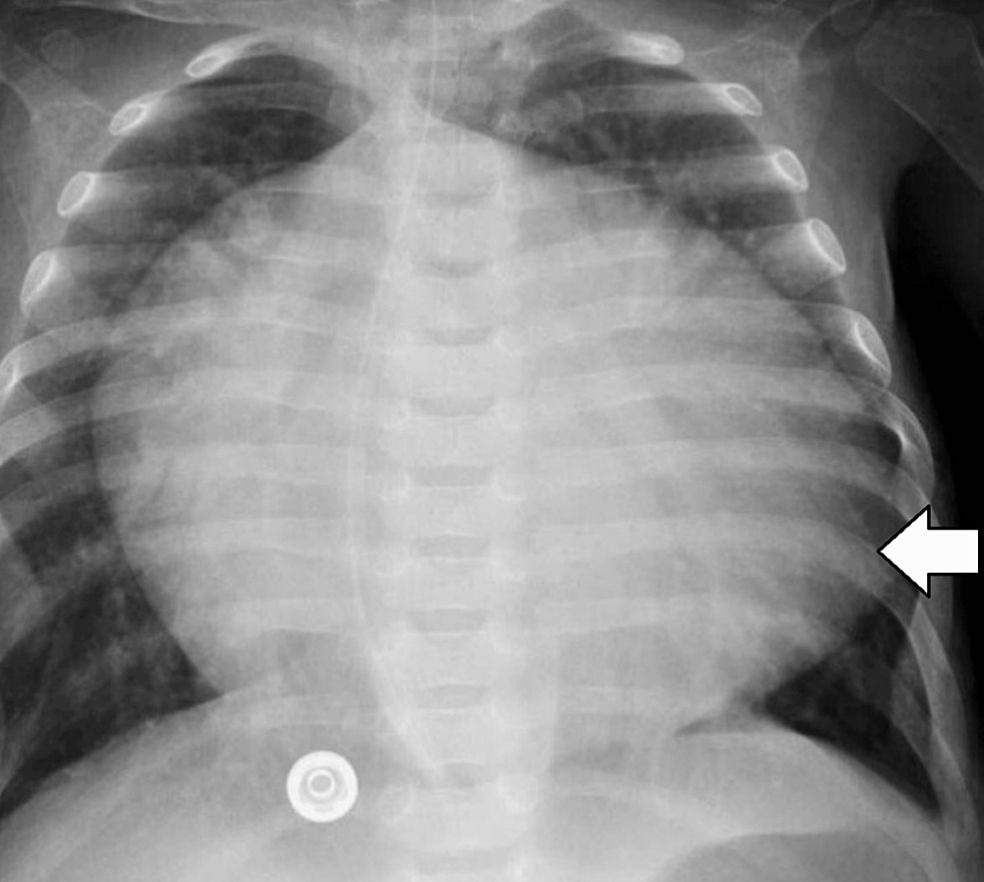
Chest X-Ray Revealing Cardiomegaly With a Narrow Vascular Pedicle and Congested Pulmonary Vasculature
